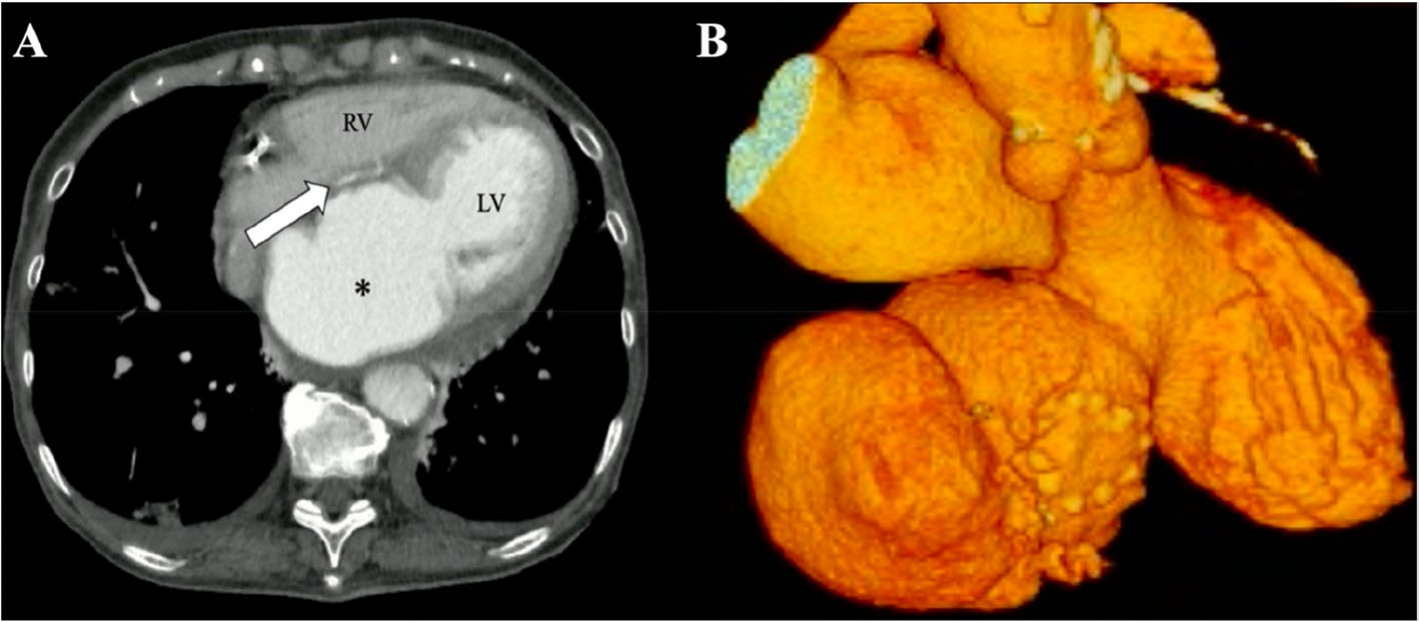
Axial (A) and 3-dimensional (B) enhanced computed tomography showed a large left ventricular septal aneurysm (star), and a patch of the right ventricle in the first operation could be seen on the anterior surface of the ventricular septal aneurysm (arrow)
